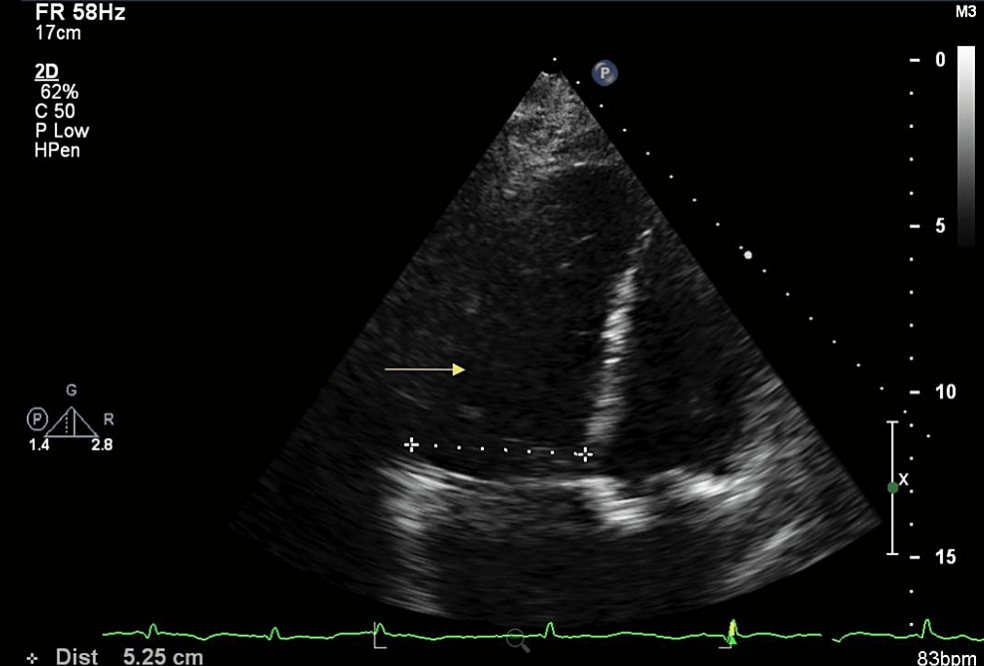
Echocardiography The yellow arrow shows the severely dilated right ventricle
